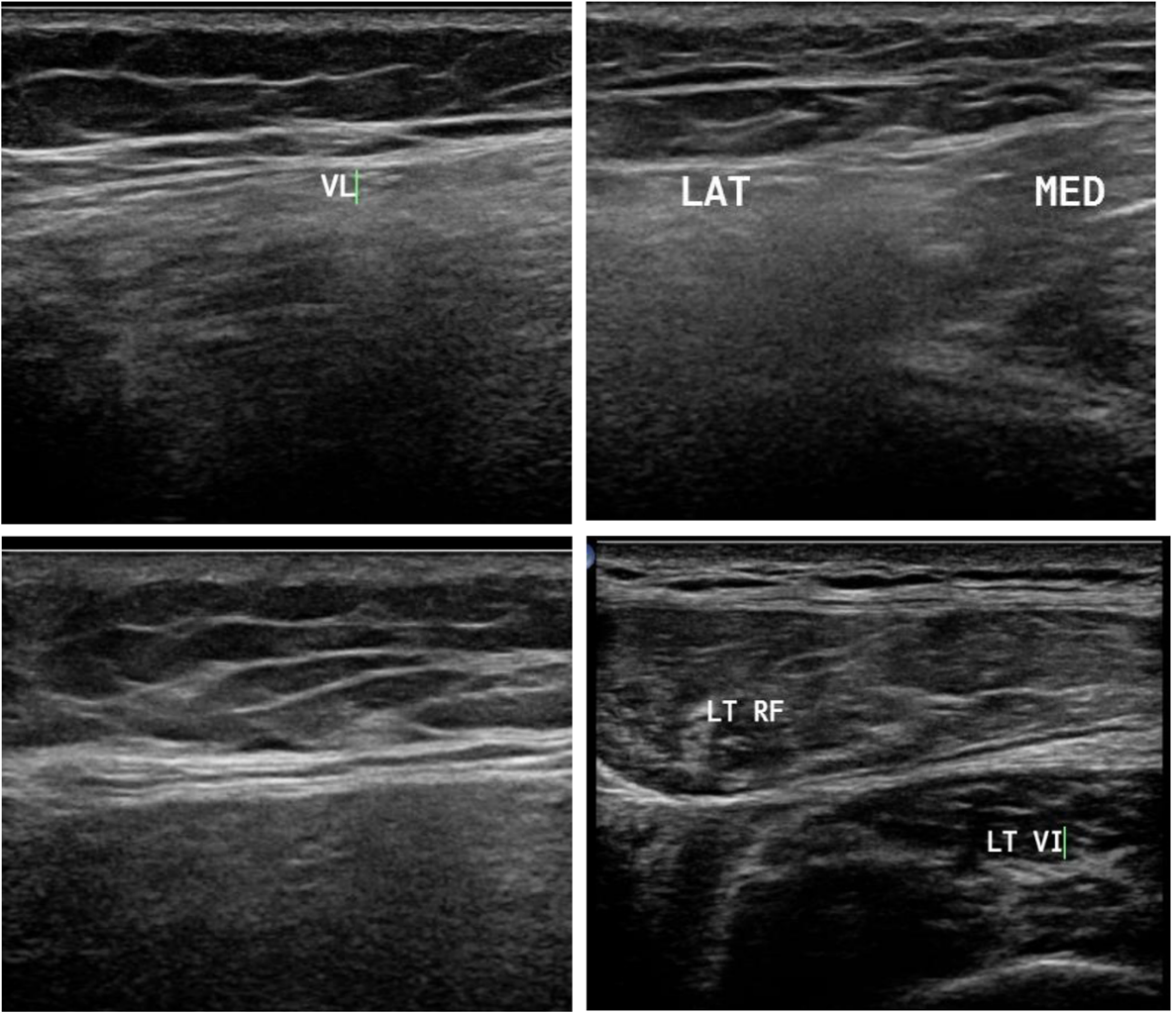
Muscle ultrasound in patient with X-linked recessive Emery-Dreifuss muscular dystrophy (EDMD) showing minimal affection in the thigh especially of the vastus lateralis (VL) and vastus intermedius (VI) and in leg selective affection of soleus
